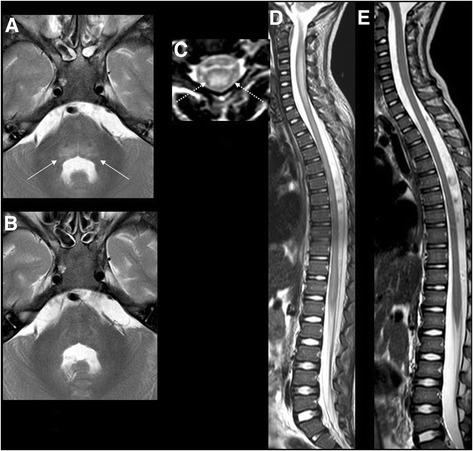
Magnetic resonance imaging (MRI) of the brain and spinal cord: a, axial T2 image at the level of the pons showing an increased signal in the tegmentum (*white arrows*), which was completely resolved in the follow up scan acquired 3 weeks later; b, sagittal and axial T2 image of the spinal cord (c, d) showing cord swelling, particularly at the cervical level (*white*, *dot arrow*), with extensive hyperintensity in the central cord (d), which was also completely resolved in the follow up images (e) acquired 3 weeks later
